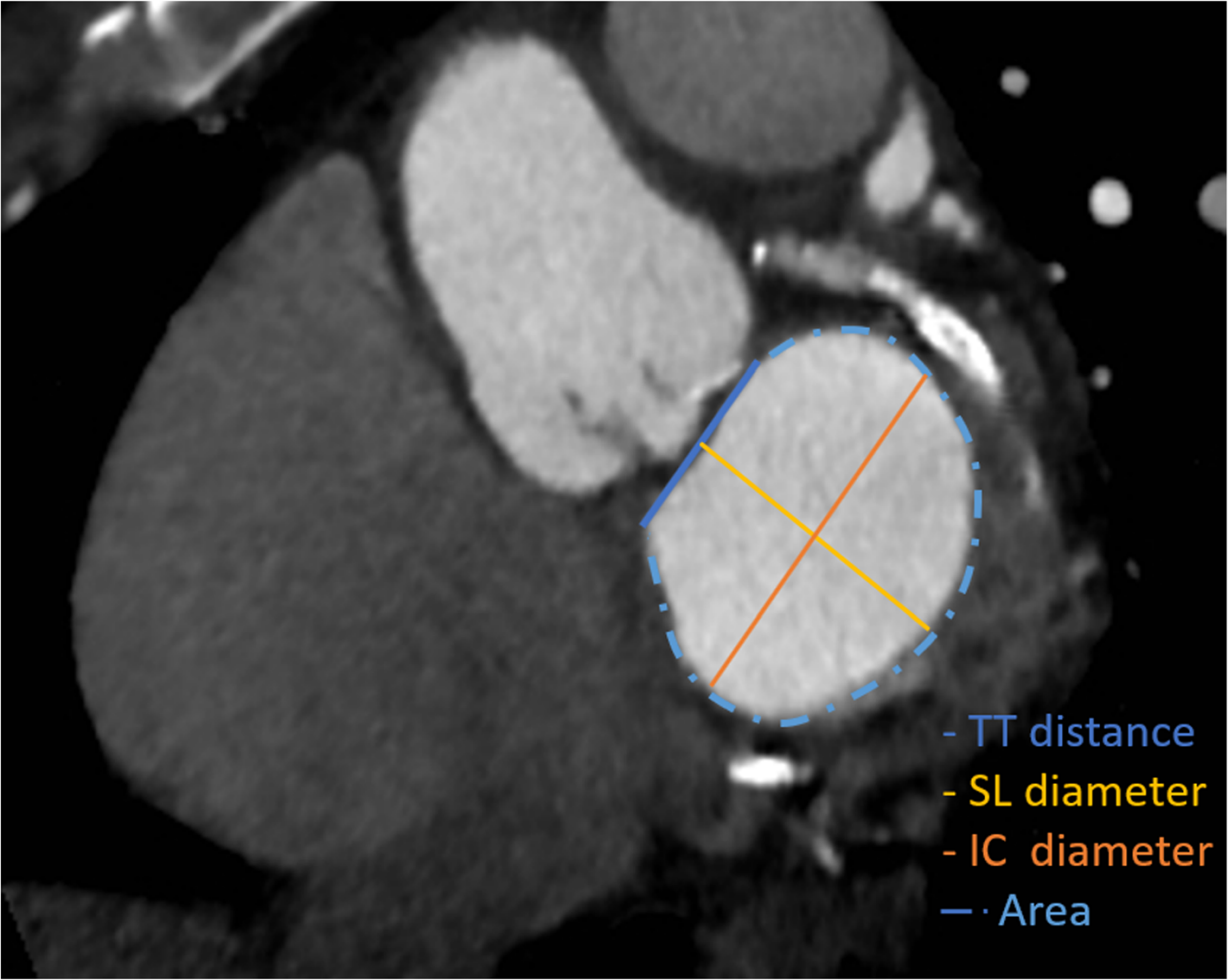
D-shaped segmentation of mitral annulus on cardiac computed tomography angiography (CCTA). Traced in blue is the trigone-to-trigone diameter, in yellow the septal-to-lateral diameter, in orange the intercommissural diameter, and the dashed light blue line plus the trigone-to-trigone diameter depict the mitral annulus
